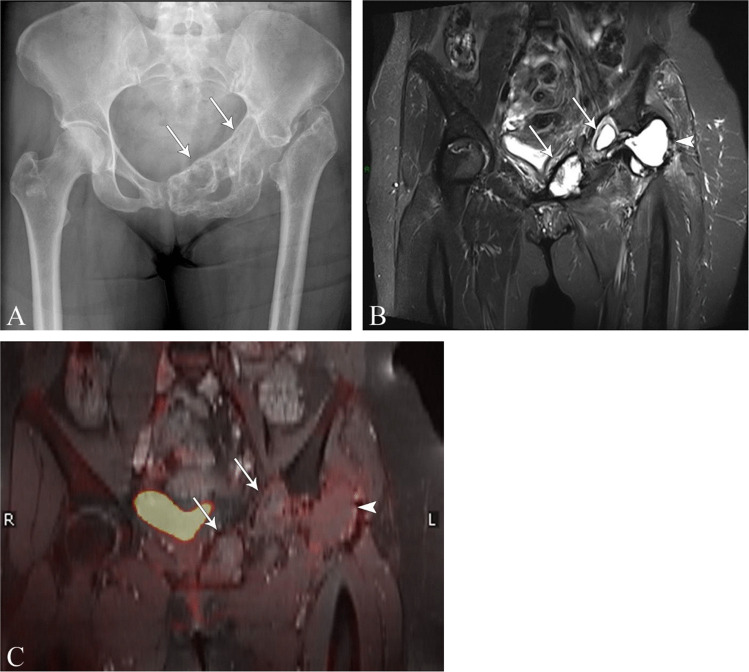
44-year-old female with pelvic Ewing sarcoma status post chemoradiation therapy. AP radiograph (A), coronal STIR MRI image (B) and coronal fused ^18^F-FDG PET-MRI image (C) demonstrate lytic/hyperintense left acetabular and superior pubic rami lesions (arrows) and small volume of fluid at the Girdlestone pseudoarthrosis (arrowheads) without significant metabolic activity, confirming treated disease
